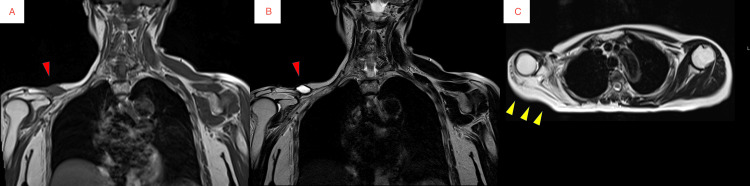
MRI of the right shoulder. (A) Coronal T1-weighted image shows high signal intensity in the right clavicle diaphysis (red arrow). (B) Coronal fat-suppressed T2-weighted image shows corresponding low signal intensity, consistent with bone marrow edema (red arrow). (C) Axial T2-weighted image demonstrates marked atrophy of the deltoid, supraspinatus, and infraspinatus muscles (yellow arrows).
